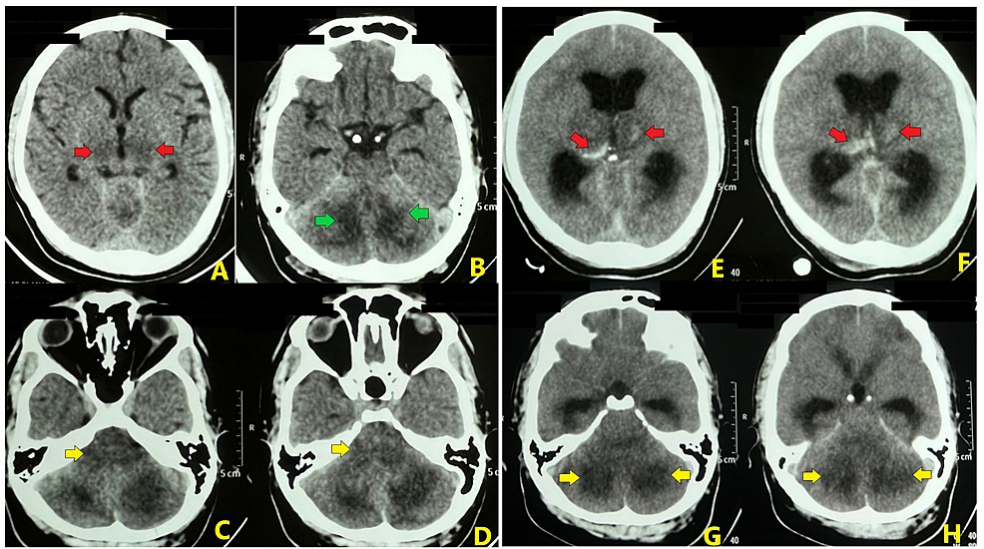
Non-contrast computed tomography of the brain demonstrating hypodensity in bilateral thalami (red arrow in A), bilateral cerebellar hemisphere (green arrow in B), and medulla (yellow arrow in C and D). Next computed tomography demonstrated intrathalamic hemorrhage (red arrow in E and F) with diffuse cerebral edema hydrocephalus and diffuse hypodensities in the cerebellar hemisphere (yellow arrow in G and H).
